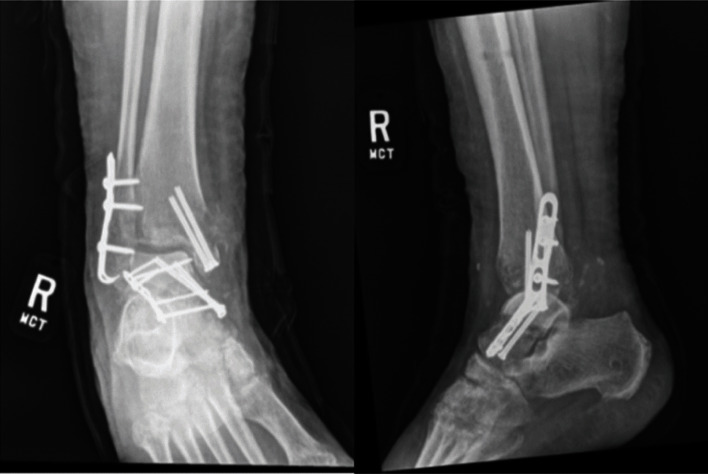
7-week follow-up; radiographs demonstrated sclerosis of talar dome and body with mild lucency of lateral dome suggestive of potential Hawkins sign.
